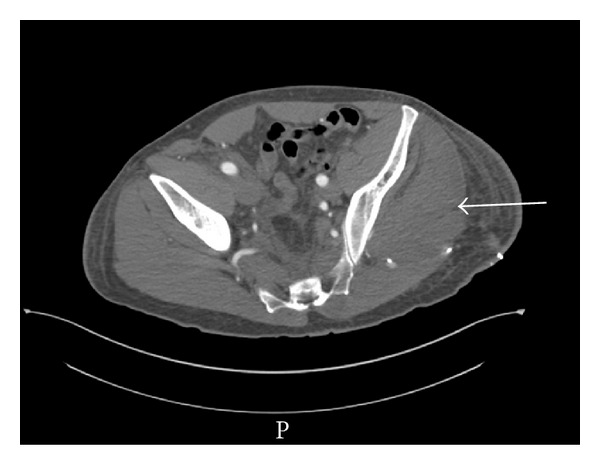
CT scan with IV contrast of the pelvis showing the swollen left gluteal region and the extravasation of contrast media.
